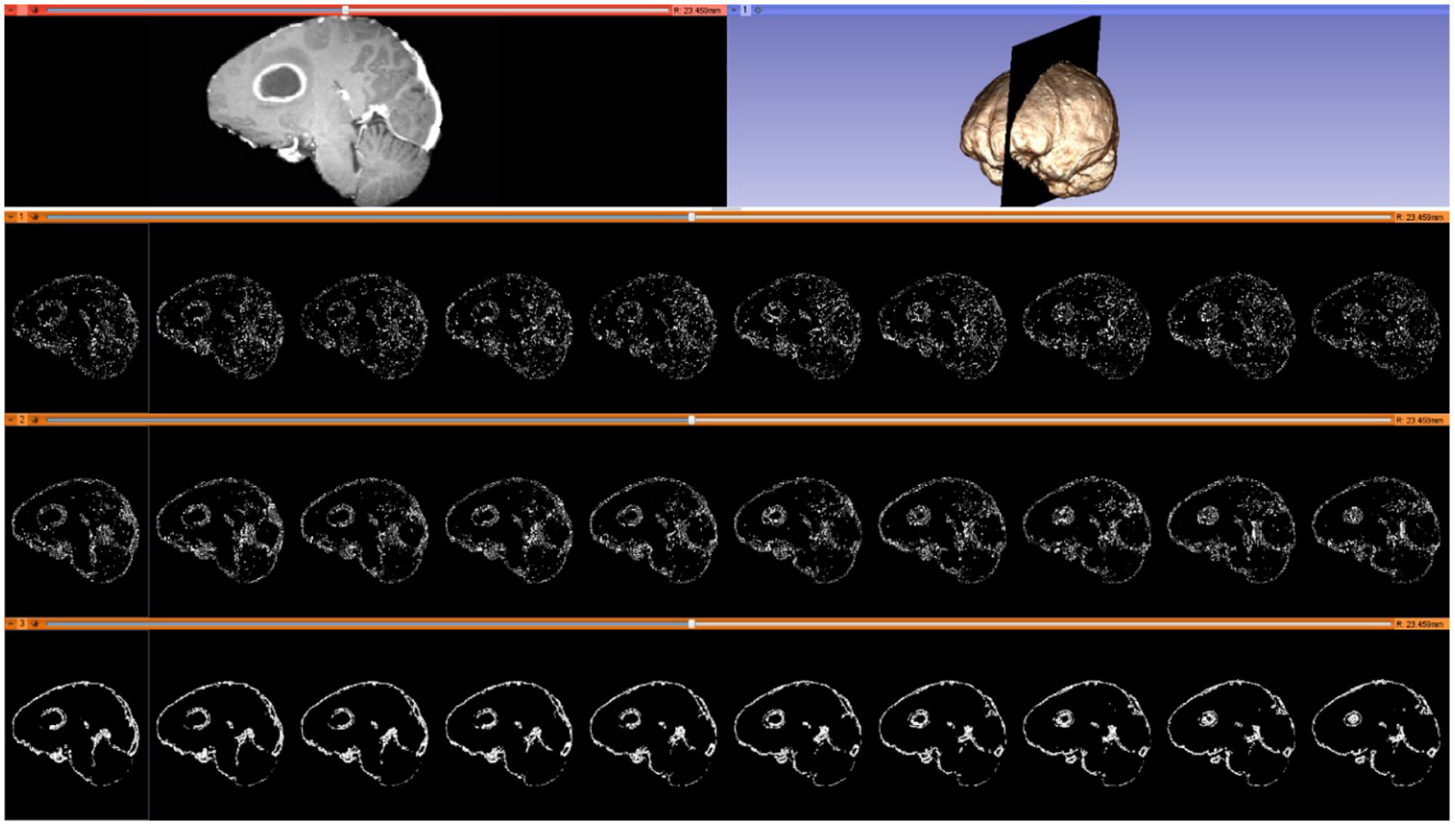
Selected blocks from an MRI volume using various connectivity patterns. Blocks are depicted on 10 consecutive sagittal slices. From top to bottom row: sagittal slice (left) and volumetric MRI rendering (right); selected blocks with a “vertex” pattern; selected blocks with an “edge” pattern; selected blocks with a “face” pattern. Number of selected blocks for all patterns: 322,060.
